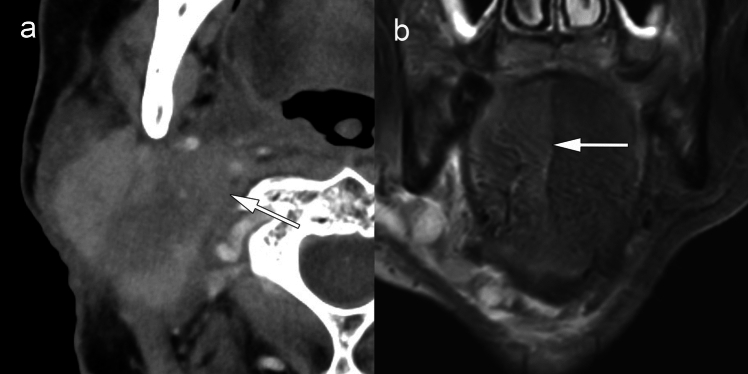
iENE Grade 3 with hypoglossal nerve invasion in a 64 year-old man with recurrent lymph node metastasis. a Contrast-enhanced CT shows level IIA extranodal extension invading the area around the posterior belly of the digastric muscle (arrow). b MRI short tau inversion recovery coronal image reveals right-sided tongue edema (arrows), indicating hypoglossal nerve paralysis due to extranodal extension. Imaging parameters: a conventional EID-CT; 512 × 512 matrix, 1 mm slice thickness. b 3 T MRI; coronal STIR sequence, 3 mm slice thickness
